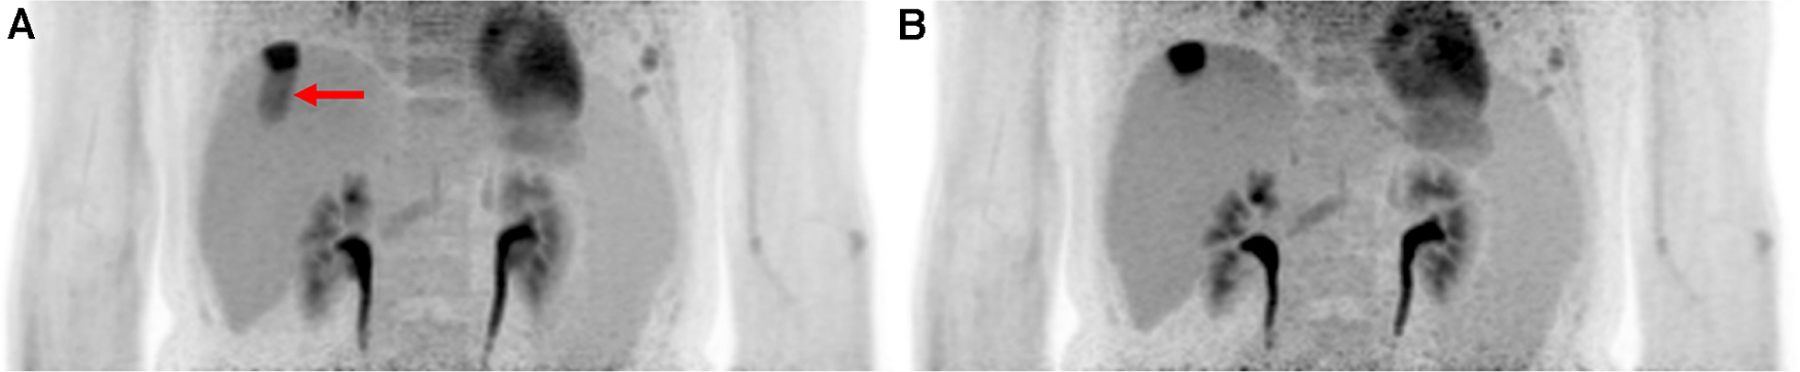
Implementing hardware-driven gating can provide drastic improvement to PET images by reducing motion artifacts. It helps improve SNR and remove motion artifacts. Abdominal PET images of the liver and kidneys are shown with (A) no gating and (B) respiratory belt gating. The red arrow shows a distinct motion artifact adjacent to an FDG-avid lesion in the ungated image.
